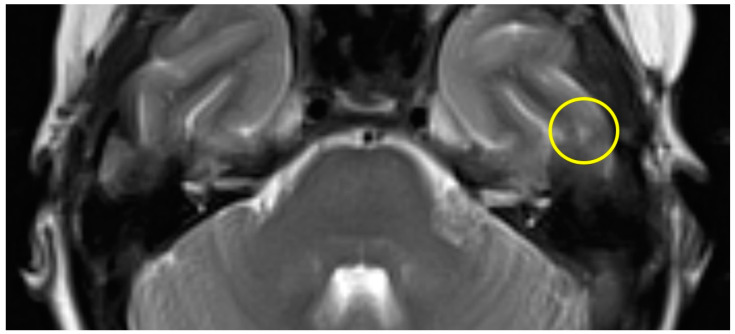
Axial T2w MR imaging of patient #3 (yellow circle = gliosis). Supplementary MR imaging can be downloaded.
